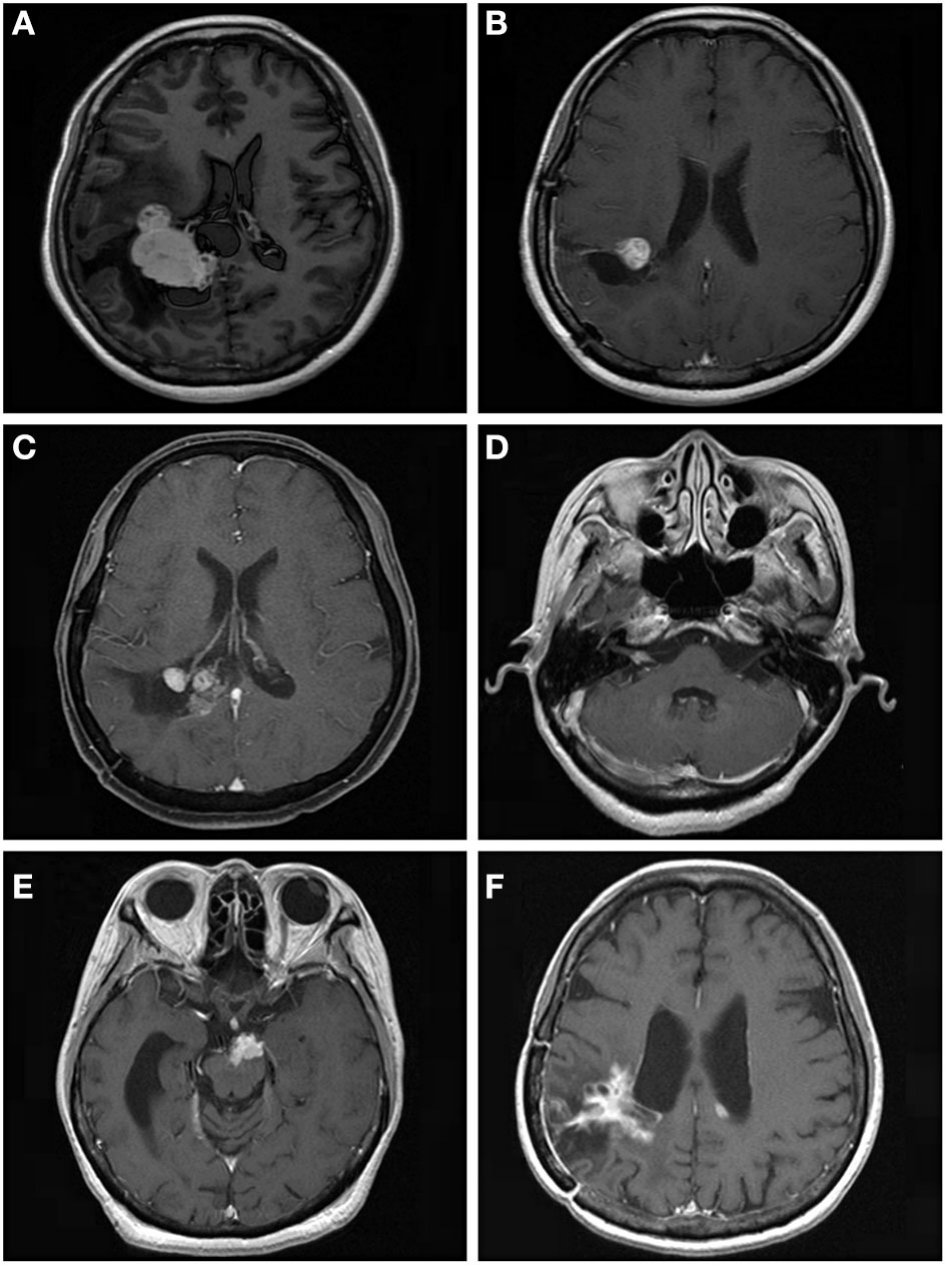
A 53.9-year-old woman with intraventricular World Health Organization (WHO) grade I meningioma received Gamma Knife radiosurgery (GKRS) (margin dose, 13.5 Gy; maximum dose, 30 Gy) as adjuvant treatment for residual tumor after surgical resection. The patient developed local tumor progression and intracranial implantation metastasis at 59.9 and 111.7 months, respectively. (A) contrast-enhanced T1-weighted magnetic resonance imaging (MRI) scans showed an intraventricular tumor. (B) Adjuvant GKRS for residual meningioma after surgical resection. (C) The patient developed local tumor progression at 59.9 months after GKRS. (D–F) The patient developed cerebellopontine angle, brainstem, and intraventricular metastasis at 111.7 months after surgical resection.
